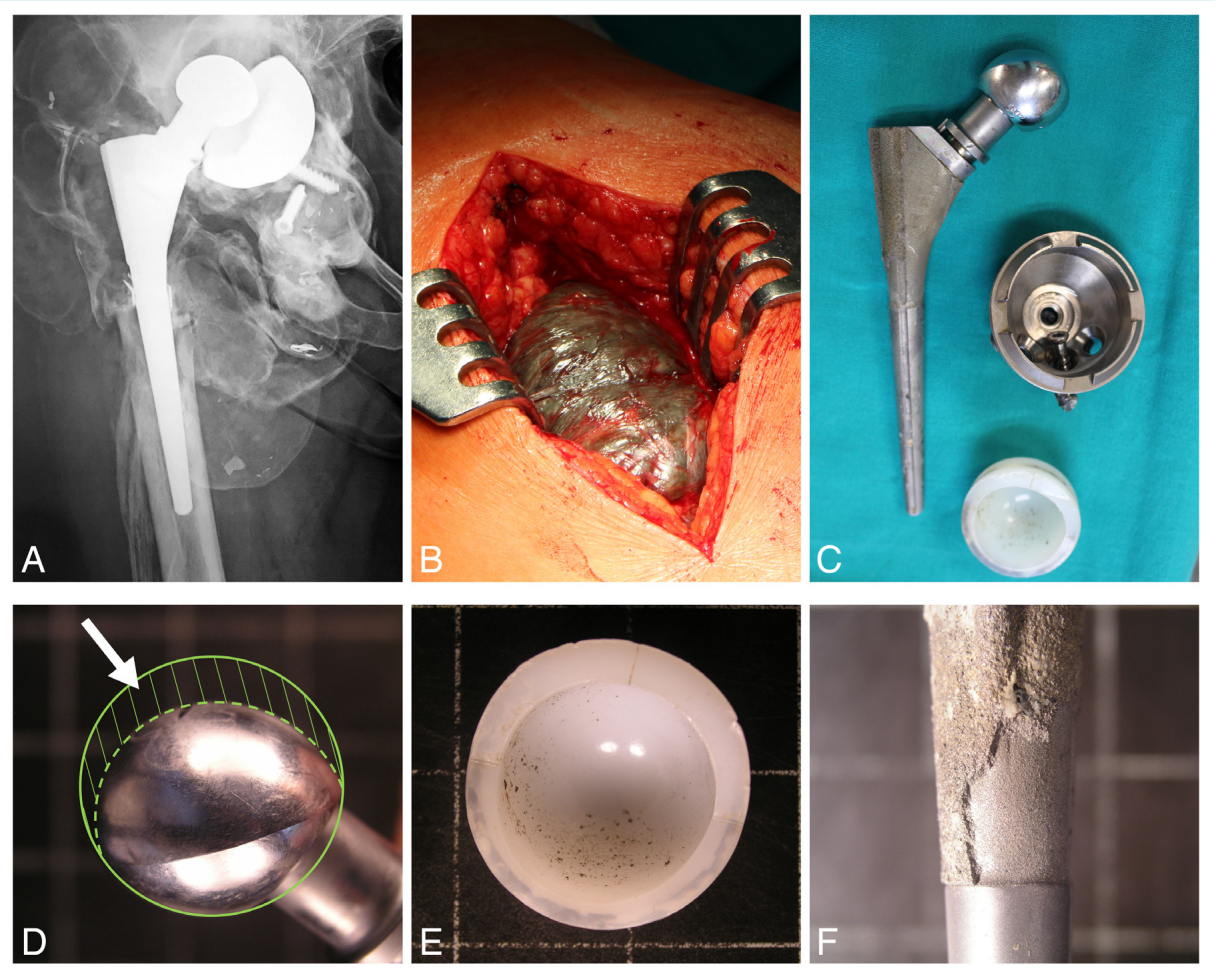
(A) Preoperative anteroposterior right hip x-ray showing acetabular cup dislocation, eccentric femoral head wear, “cloudy bubbles,” and pseudotumor formation; (B) intraoperative image after a direct lateral approach to the right hip showing extensive metallosis; (C) intraoperative image of the extracted endoprosthetic components; (D) eccentric wear of the femoral head (posterior view, arrowhead pointing approximately at the worn part of the femoral head); (E) metal debris particles embedded in the acetabular polyethylene – most of the particles are embedded in the part of the liner that was not in contact with the worn femoral head; (F) damaged titanium porous coating on the extracted femoral stem.
